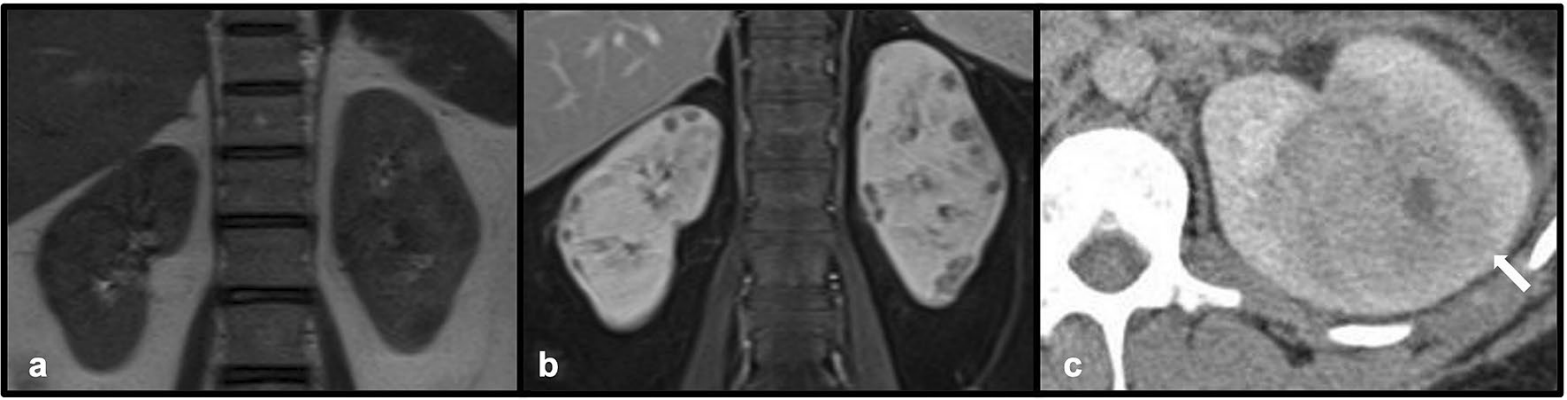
Coronal T2 (a) and T1-VIBE post-gadolinium (b) images demonstrate numerous small, hypointense bilateral renal cortical defects in a patient with renal-biopsy-proven GPA. Axial post-contrast CT( c) in another patient demonstrates a heterogeneous mass in the left kidney with central areas of low attenuation (white arrow) suggestive of necrosis. Biopsy confirmed the presence of non-caseating granulomas consistent with GPA
